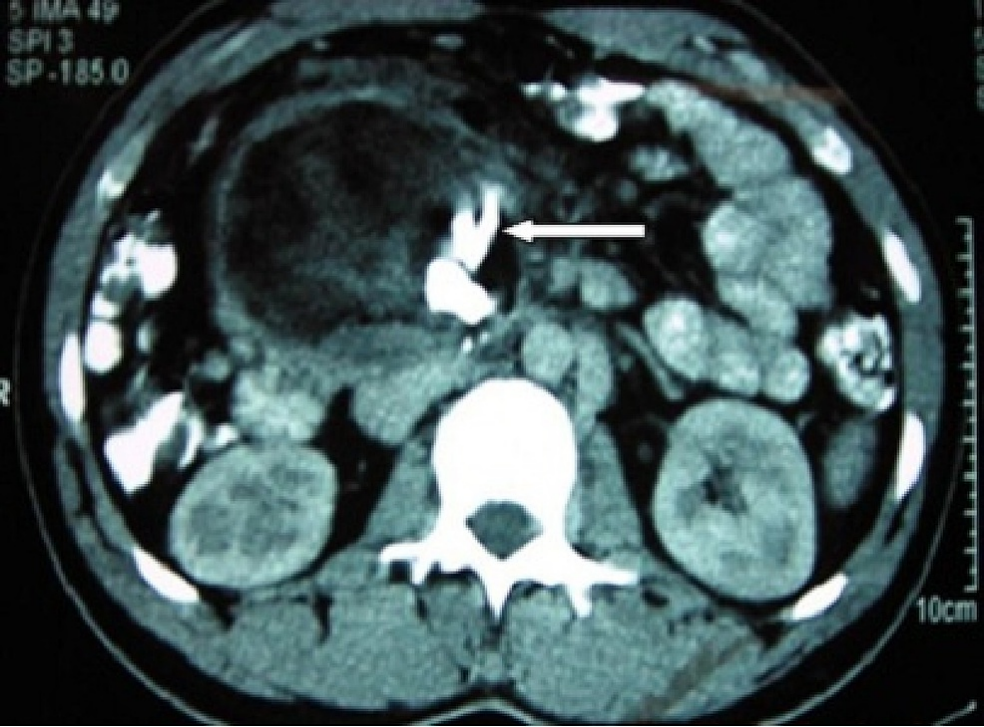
Contrast-enhanced computed tomography of the abdomen Contrast-enhanced computed tomography of the abdomen revealed an 8.6 cm x 7.7 cm heterogeneous soft tissue density mass from the retroperitoneum with calcification within (arrow).
